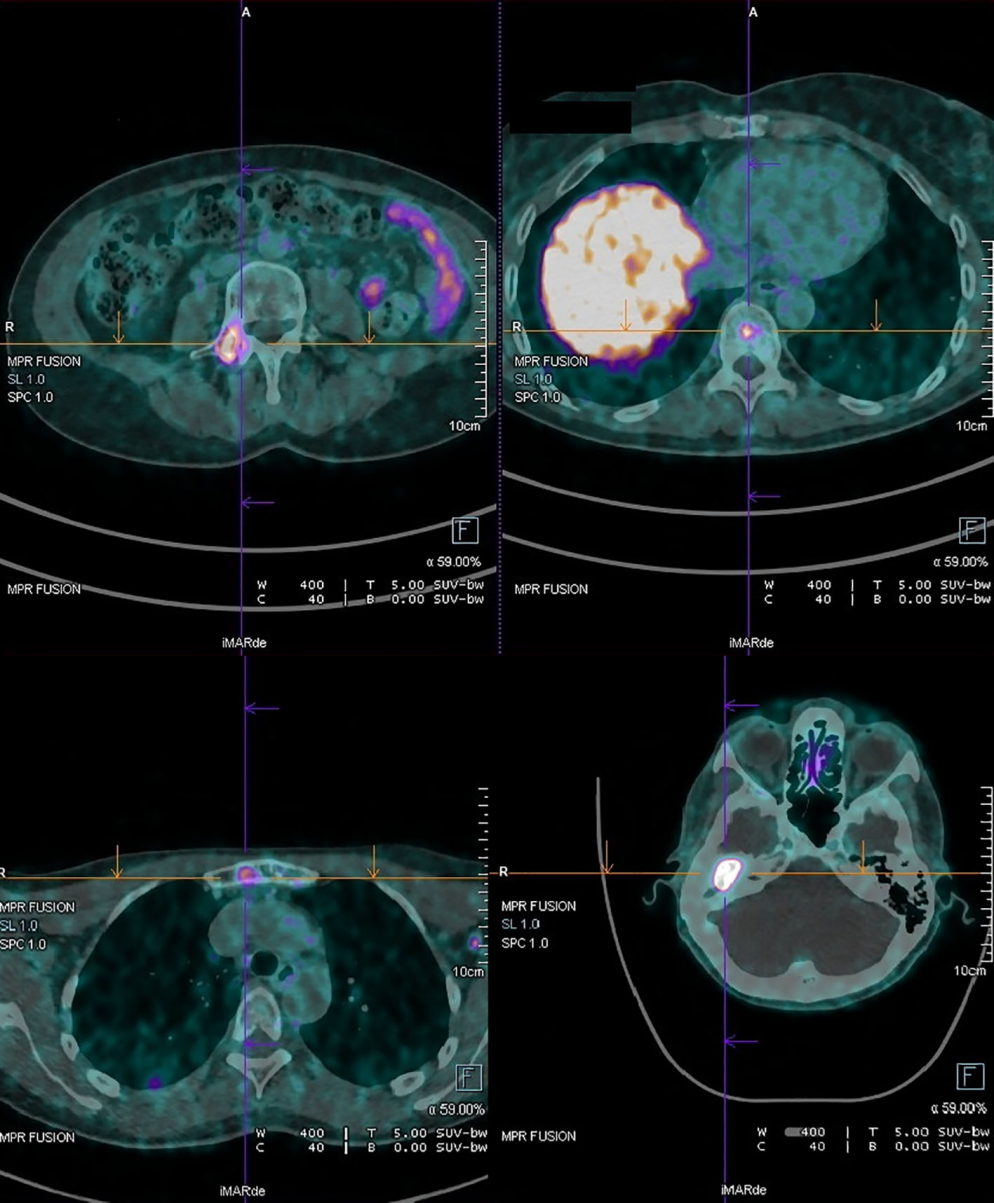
68Ga-DOTATATE PET/CT scan performed in July 2024 in case 3. It demonstrated an increase in the expression of somatostatin receptors in the right middle ear and skeletal sites (sternum, vertebrae).
